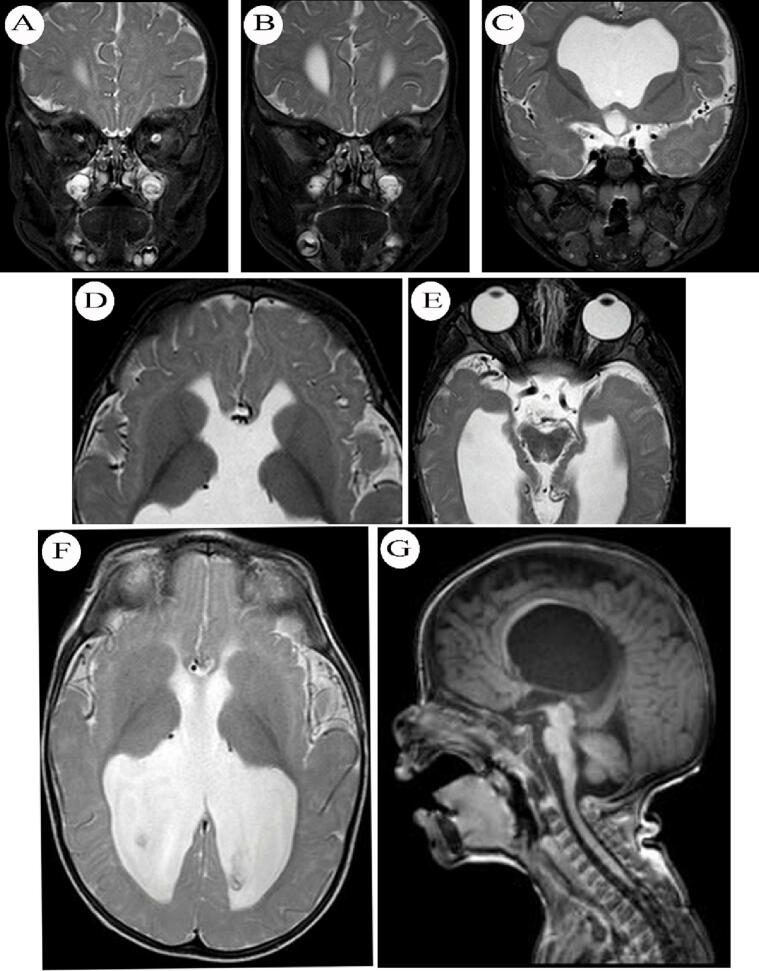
Brain MRI of the child presented in Box 1. Images A, B, and C are T2 coronal sections showing hypoplastic optic nerves and chiasma with a normal-sized pituitary gland. Image D is an axial T2 section showing box-like dilated frontal horns of the lateral ventricles. Image E is another T2 axial section showing hypoplastic optic nerves and chiasma and dilated lateral ventricles. Image F is an axial T2 film showing dilated lateral and third ventricles, colpocephaly, and absent septum pellucidum. Image G is a sagittal T1 section showing dilated lateral ventricle and thinned out hypoplastic corpus callosum.
